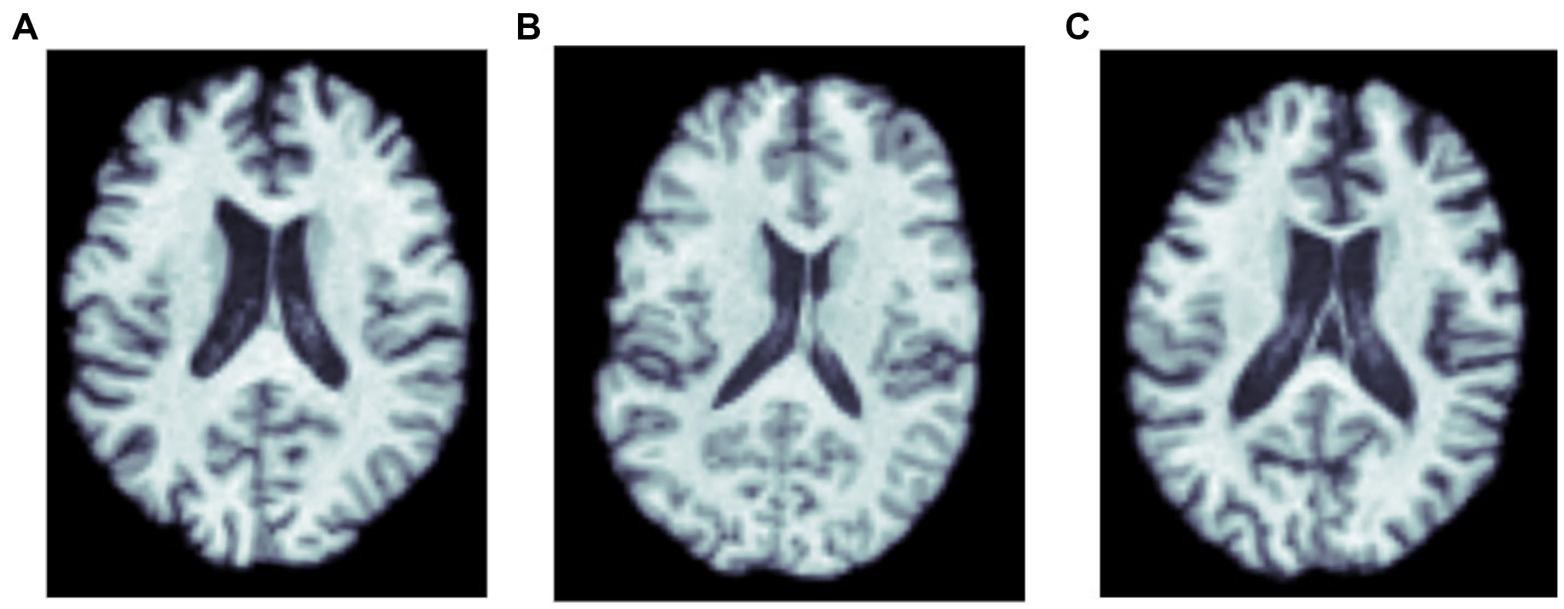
MRI scans of three amyloid positive participants: (A) a cognitively normal control, and participants diagnosed with (B) MCI, and (C) dementia.
